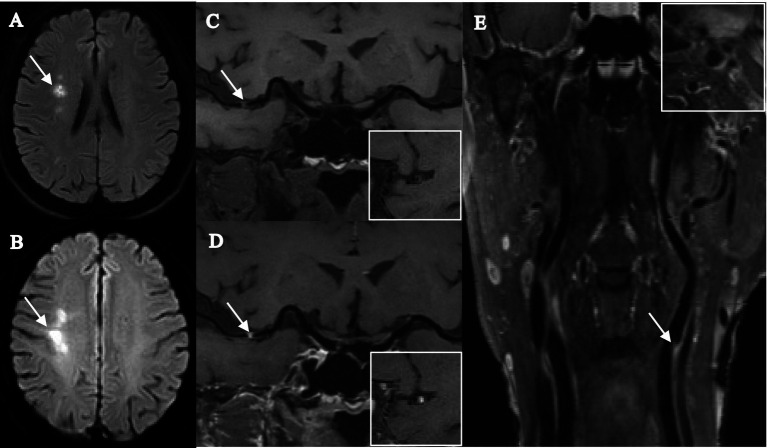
Female, 68 years old, was admitted to the hospital with numbness in the left limb, and stroke recurrence occurred at the 4-month follow-up. (A) DWI images before recurrence shows a patchy hyperdense shadow visible in the right corona radiata (arrow). (B) DWI images after recurrence shows a clumpy hyperdense shadow visible in the right centrum semiovale (arrow). (C,D) T1-SPACE images before and after enhancement show the presence of plaque in the M1 segment of the right middle cerebral artery (arrow), with high plaque burden, significant enhancement after enhancement, and severe stenosis. (E) T1-SPACE image of the extracranial carotid artery shows the presence of plaque at the beginning of the left internal carotid artery (arrow).
